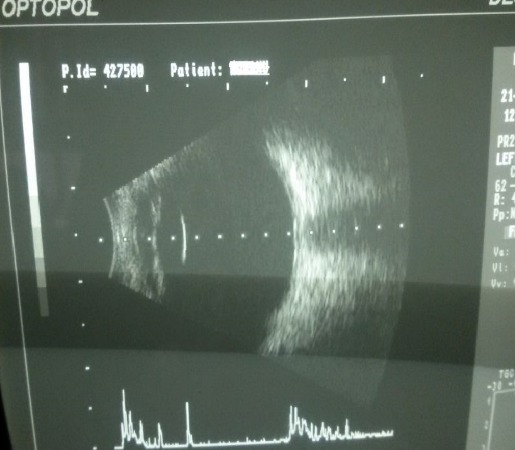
B-scan ultrasonography OD
